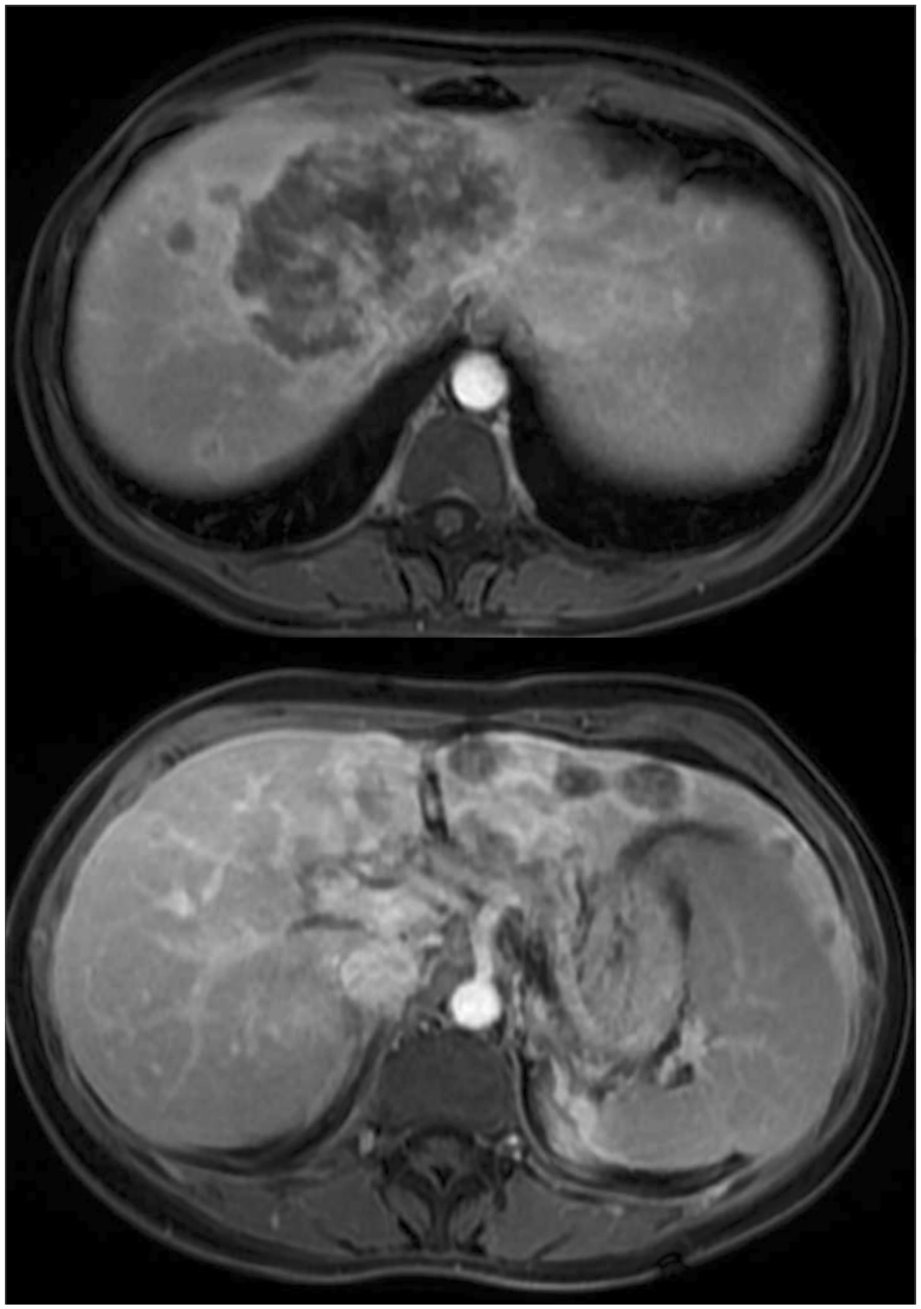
Magnetic resonance imaging showed a multinodular lesion with a predominantly tumor in the central liver with cava vein invasion.
